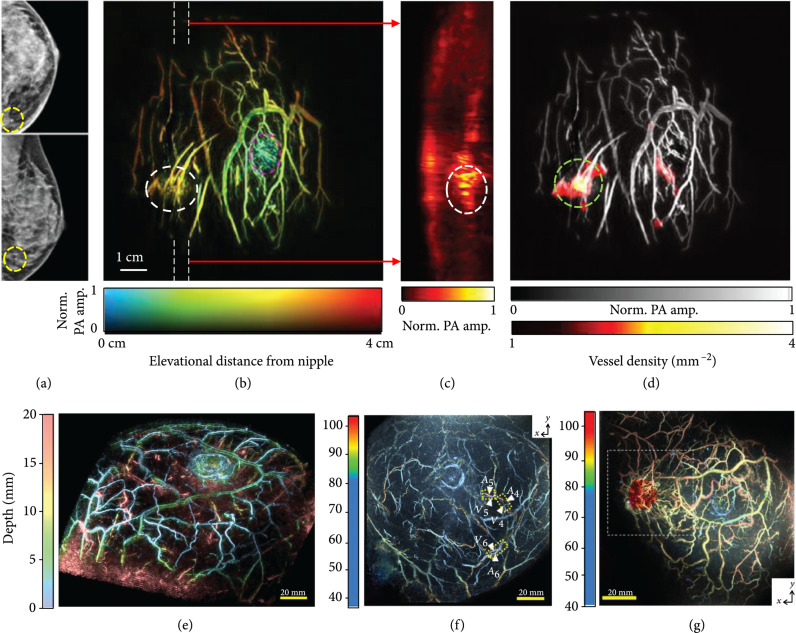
PACT of human breasts. (a) X-ray mammograms of an affected breast [94]. (b) Depth-encoded angiogram of the affected breast acquired by SBH-PACT. The breast tumor is identified by a white circle; the nipple is marked by a magenta circle [94]. (c) Maximum amplitude projection (MAP) images of the thick slice in sagittal planes marked by white dashed lines in (b) [94]. (d) Automatic tumor detection on vessel density maps. Tumors are identified by green circles. Background images in gray scale are the MAP of vessels deeper than the nipple [94]. (e) Depth-encoded 3D PACT image of a healthy breast [95]. (f) Evaluation of the S-factor in a healthy breast, where the color represents the measured S-factor [96]. (g) A fusion image of the S-factor and 3D-US images (red color) [96].
